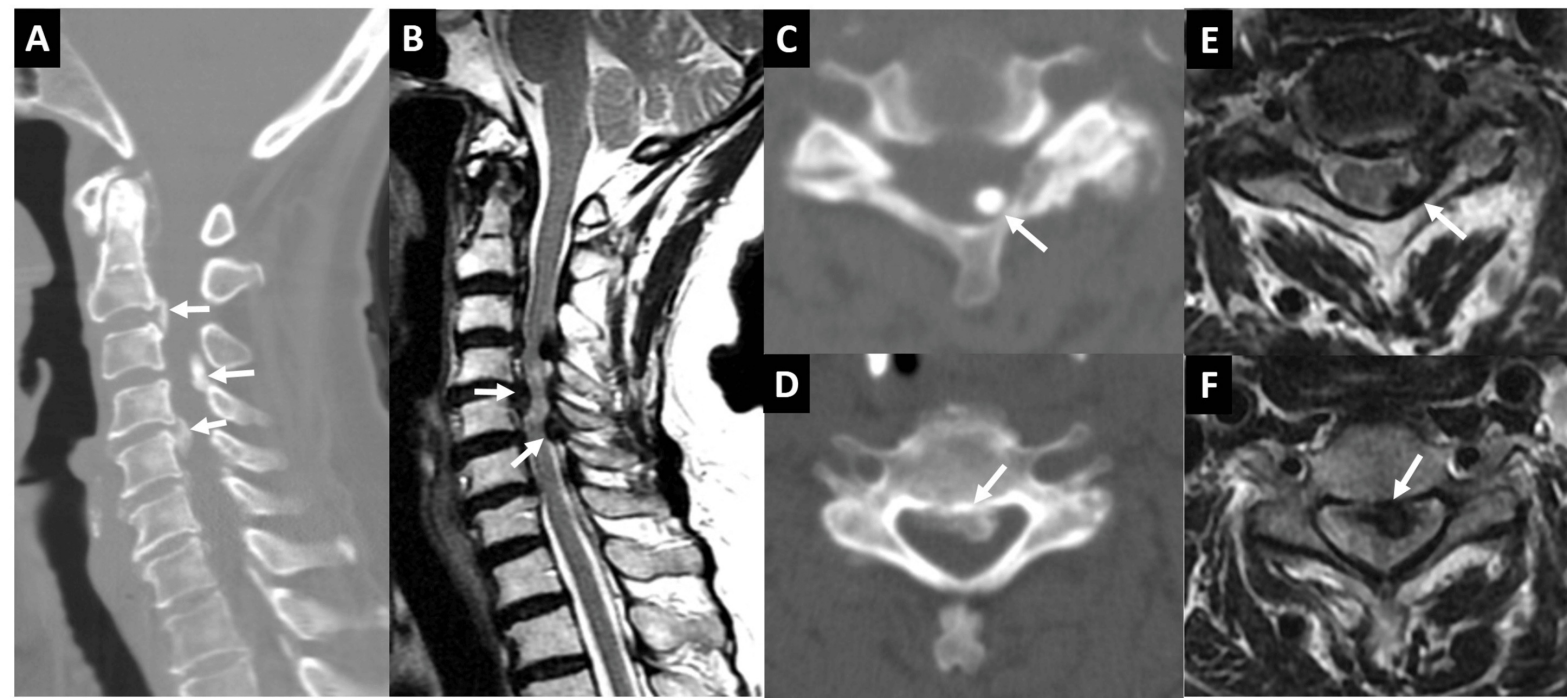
CT and MRI images of the cervical spine (A) A sagittal CT image shows protruding discs and calcified CLF, indicated by white arrows. There is a small calcific deposit in the atlantoaxial joint region. (B) A sagittal T2-weighted MRI reveals hypointense lesions arising from the discs, PLL, and CLF. An abnormally high signal intensity in the spinal cord implies myelopathy. White arrows indicate the protruding disc and CLF. (C, D) Axial CT images at C3-C4 (C) and C4-C5 (D) reveal narrowing of the spinal canal caused by the calcified CLF and calcified protruding disc, respectively, marked by white arrows. (E, F) Axial T2-weighted MRI at C3-C4 (E) and C4-C5 (F) reveal hypointense lesions arising from the discs and CLF. These lesions compress the spinal cord from anterior and posterior aspects, respectively, indicated by white arrows. CLF: cervical ligamentum flavum; PLL: posterior longitudinal ligament
